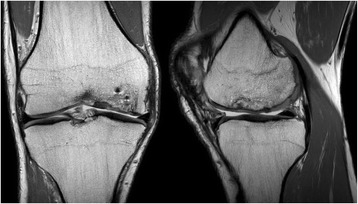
Post-Treatment PD weighted Coronal andSagittal MRI imaging at 18 months indicating persistent articular cartilage regeneration at the site of the osteochondral defect
